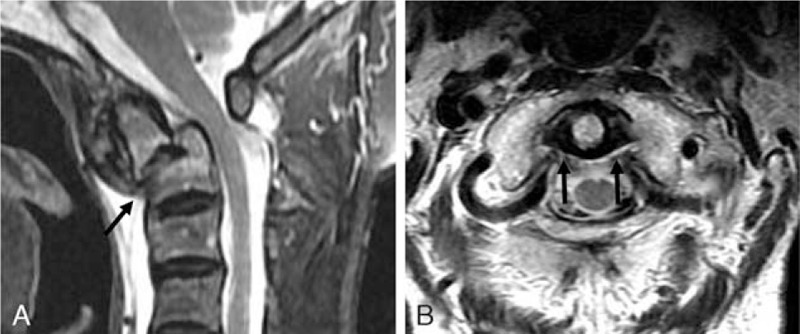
Sagittal magnetic resonance imaging (a) revealed the anteroinferior displacement of the type IIA dens fracture (dark arrow) (according to the Anderson and D’Alonzo classification) without spinal cord compression. Axial magnetic resonance imaging (b) revealed an intact transverse atlantal ligament (dark arrows).
